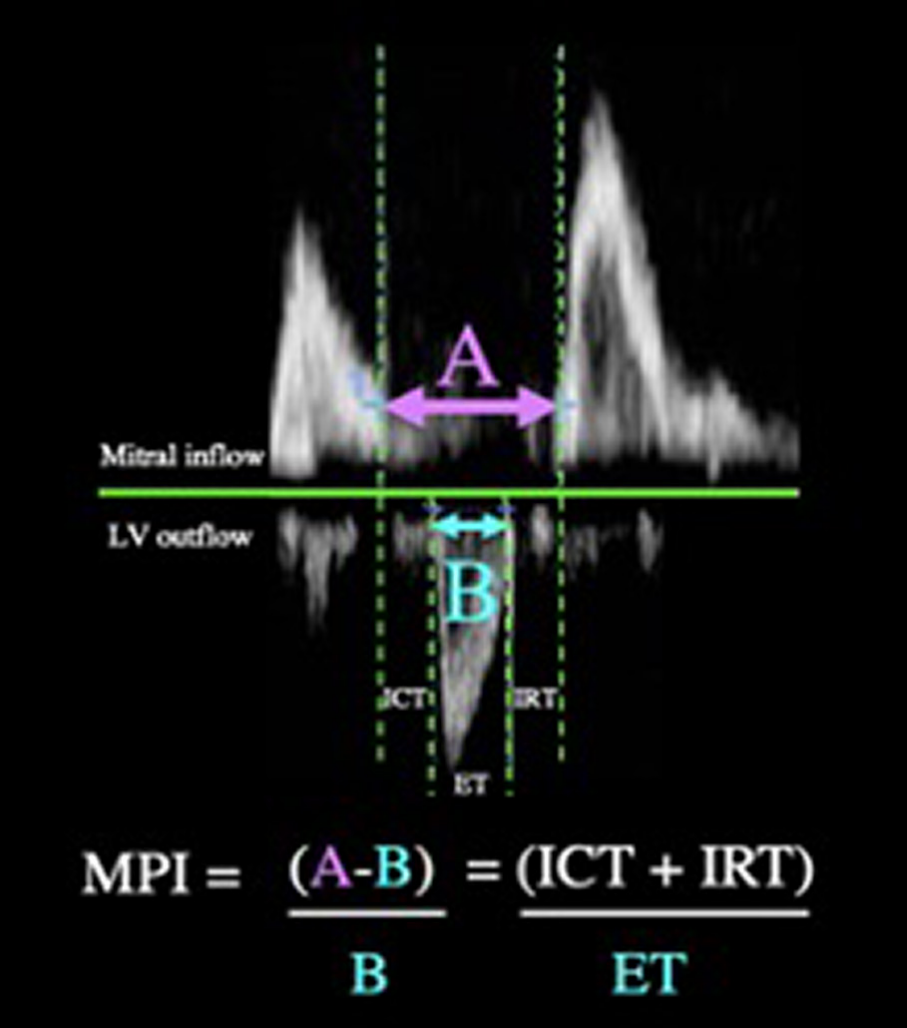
Representative image of MPI analysis from Doppler tracings of mitral inflow and LV outflow. A = time between filling periods; B = ejection time (ET). The sum of is isovolumic contraction time (ICT) and isovolumic relaxation time (IRT) can be obtained by the subtraction of B from A. Image is not to scale for the purpose of depicting the analysis graphically
